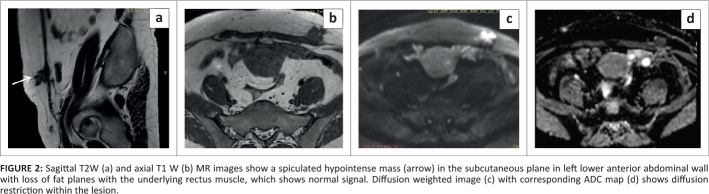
Sagittal T2W (a) and axial T1 W (b) MR images show a spiculated hypointense mass (arrow) in the subcutaneous plane in left lower anterior abdominal wall with loss of fat planes with the underlying rectus muscle, which shows normal signal. Diffusion weighted image (c) with corresponding ADC map (d) shows diffusion restriction within the lesion.
